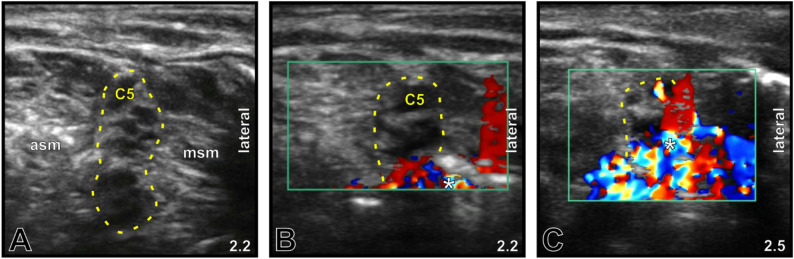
Color Doppler ultrasound-guided PNC tip correction at the interscalene brachial plexus in a fixed cadaver. A B-mode image showing the interscalene brachial plexus (*yellow dotted line, C5*) located between the anterior (*asm*) and the middle scalene muscle (*msm*). The ultrasound imaging depth [cm] is shown in the lower right corner. B Color Doppler mode image demonstrating an improvable CDS adjacent to the caudal portion of the brachial plexus. The *white asterisk* indicates the site of initial hydrodissection and the CDS, corresponding to the PNC tip position. C Color Doppler mode image after PNC retraction by 0.5 cm, showing a distinct and reliable CDS adjacent to the cranial portion of the brachial plexus, which is consistent with optimized PNC tip positioning
